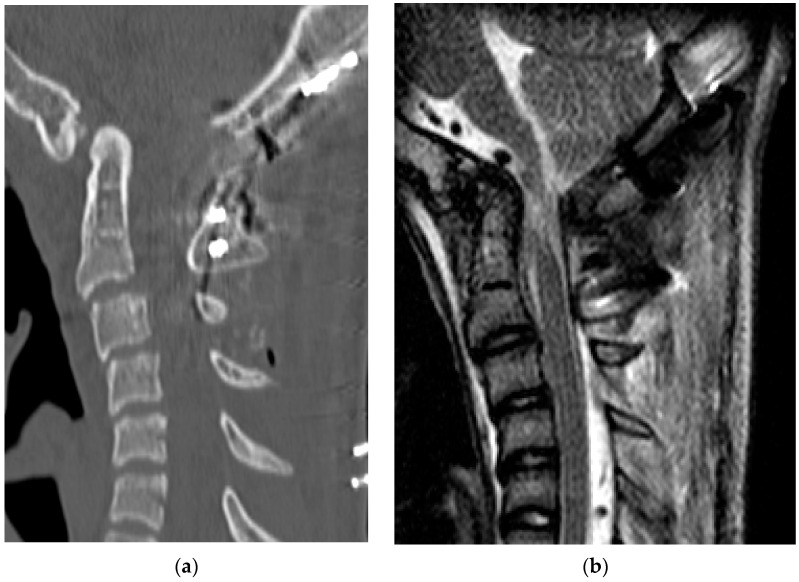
Preoperative images: (a) sagittal view of a computed tomography scan; (b) sagittal view of the T2-weighted magnetic resonance images.
